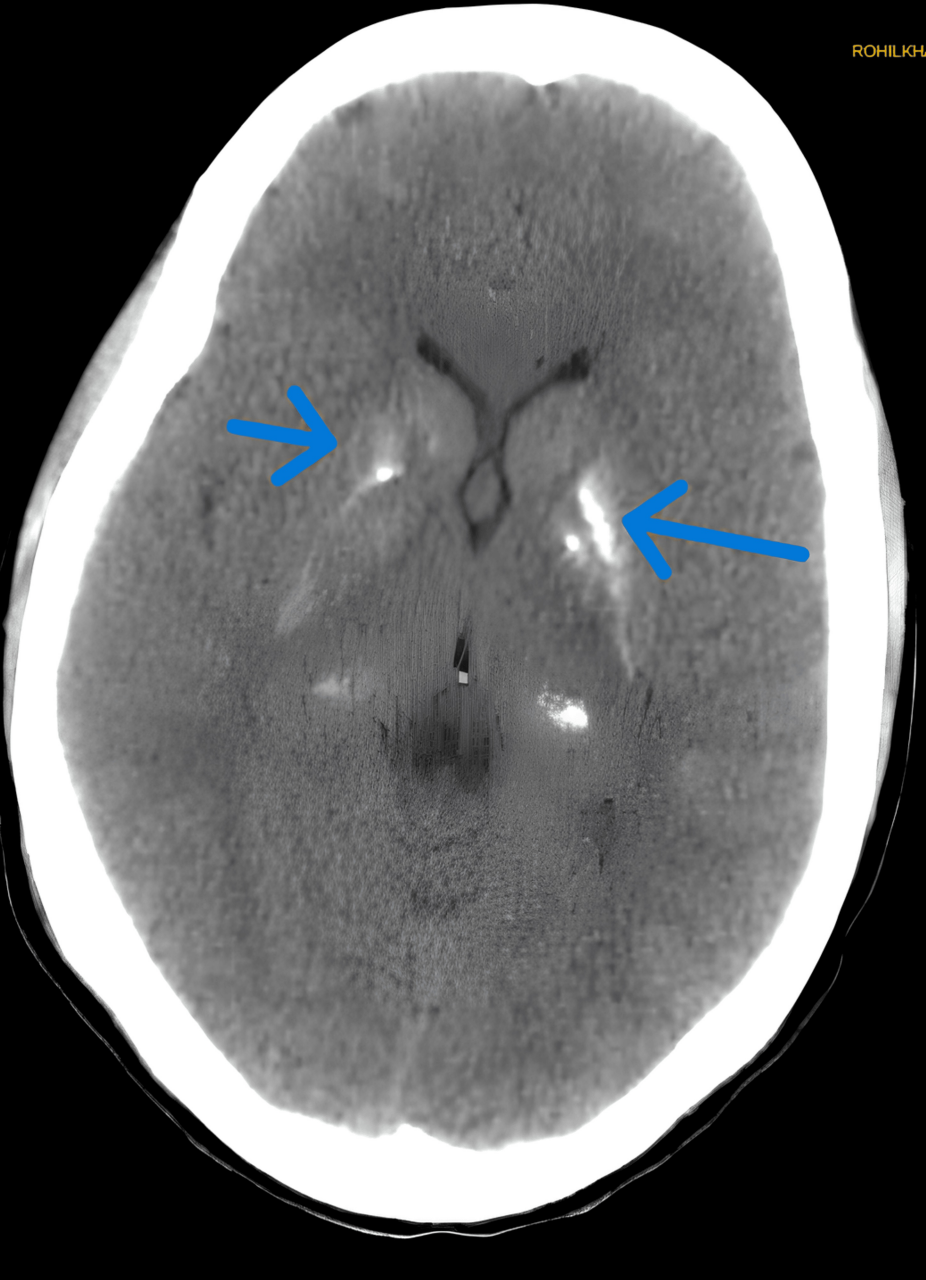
CT Brain showing hyperintense signal in bilateral basal ganglia and thalamus. Blue arrow pointing towards hyperintense signal in bilateral basal ganglia.
